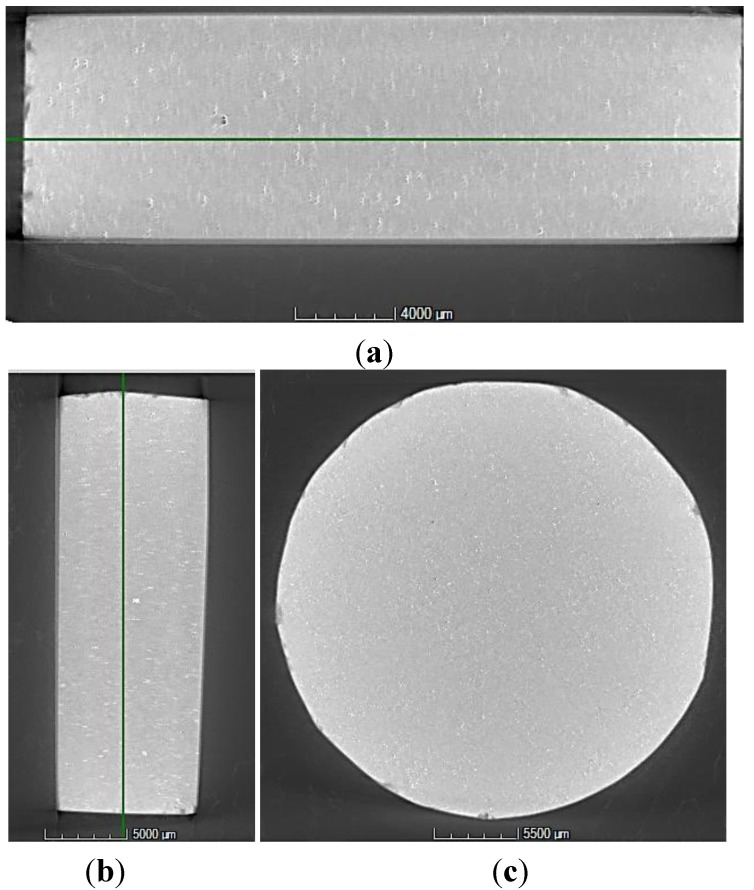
Computed tomography (CT) images according to three orthogonal planes for Sample S3: (a) *xy* plane; (b) *yz* plane; and (c) *xz* plane.
